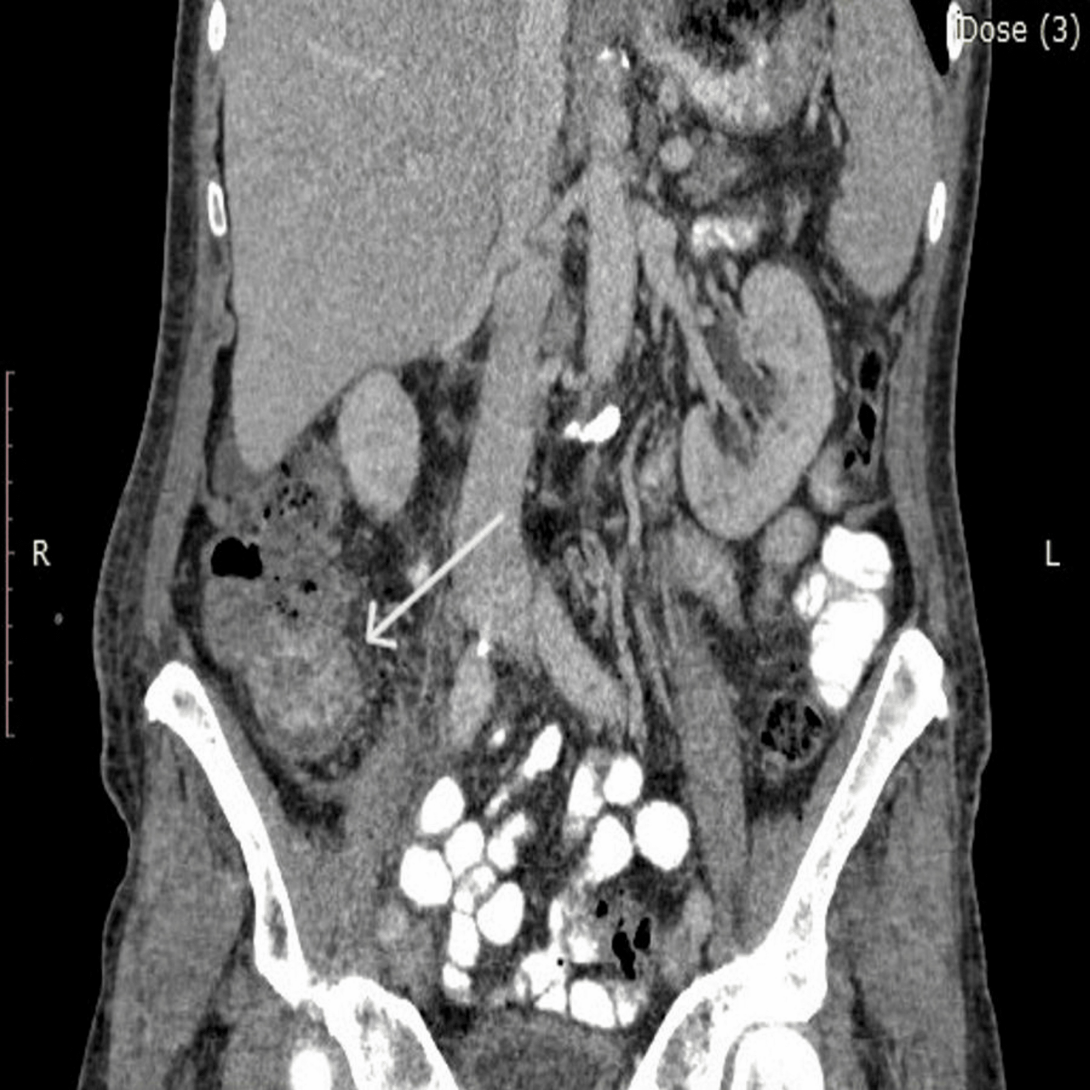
Abdominal CT scan (axial and coronal) showing the lesion CT: computed tomography
